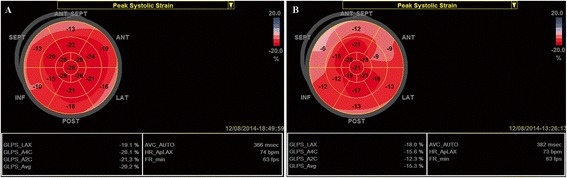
Left ventricular global longitudinal strain (GLS) measured by speckle tracking echocardiography in two asymptomatic patients with severe AS, a similar degree of concentric LVH and LVEF > 60%. Reduced values of longitudinal deformation in the basal LV segments are observed in the first patient, but with a GLS value within normal range (−20%) (A). Impaired GLS (−15%) was found in the second patient, with more severely reduced values of longitudinal deformation in the basal segments (B). Stress echocardiography was performed in both patients. The second patient experienced dyspnea at a low level of exercise while the first remained asymptomatic. Angiography in the second patient revealed no significant coronary lesions.
